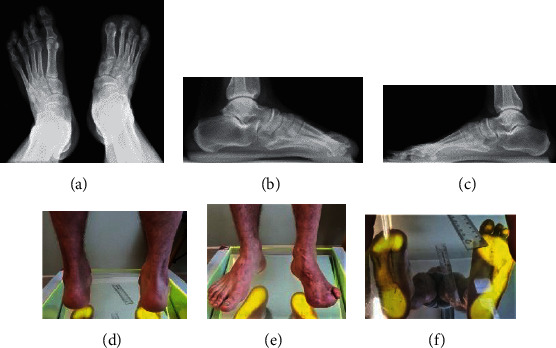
a–f) Results of imaging tests (X-ray) and podoscopic tests: patient no. 11. (a) Dorsal-plantar foot X-ray: fatigue fracture of the III metatarsal bone, amputation of toes I–IV in the left foot. (b) Lateral X-ray: amputation of toes I–IV, overload in the area of ankle joints, and talonavicular. (c) Lateral X-ray: overload in the area of ankle joints and talonavicular. (d) Posterior photo: ankle joint and hindfoot evaluation. (e) Anterior picture of the feet: assessment of toe positioning and deformation. (f) Plantar photo on the podoscope: assessment of plantar part of the foot and height measurement of the longitudinal arch.
